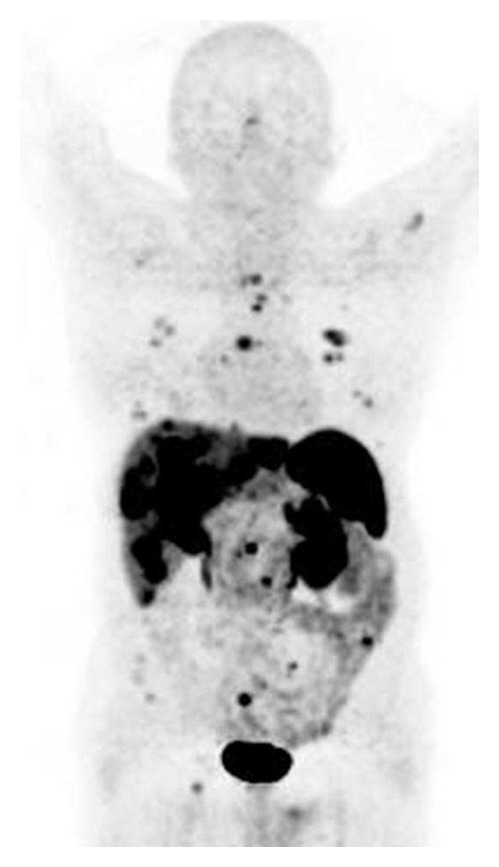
^68^Ga-DOTATOC PET/CT: liver, lung, lymph node, and bone metastases from NET of unknown origin.
